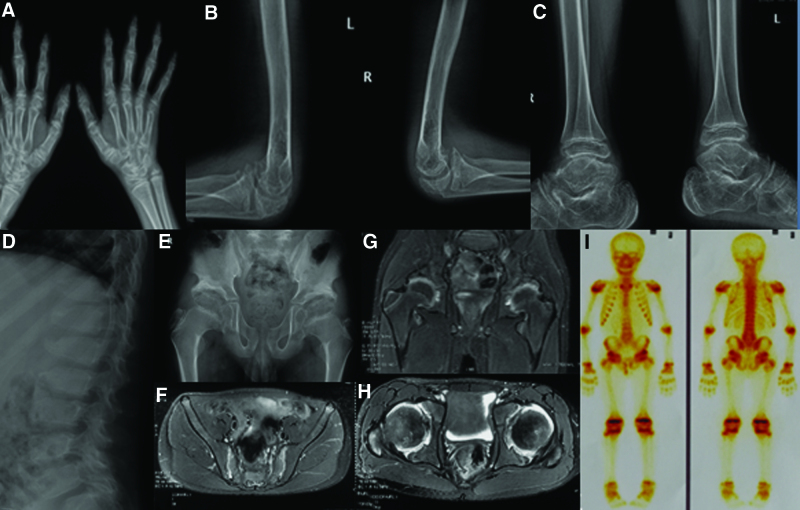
Imaging results of the patient. (A) X-ray of the hands shows dilatation of the interphalangeal joints; (B) elbows show underglazing of the articular surface and chondrodysplasia; (C) ankles show bilateral narrow joint spaces and incomplete joint surfaces; (D) spine shows extensive vertebral body compression; (E) pelvis shows a flat acetabulum on both sides, with irregular edges and sclerosis, and poor growth of the femoral epiphyses. Magnetic resonance imaging of the (F) sacroiliac joint shows bilateral sacroiliac arthritis on arthroscopic surface evaluation; (G and H) hip shows inflammatory changes in both hip joints and joint cavity effusion. (I) Whole-body bone scan reveals multiple inflammatory changes.
